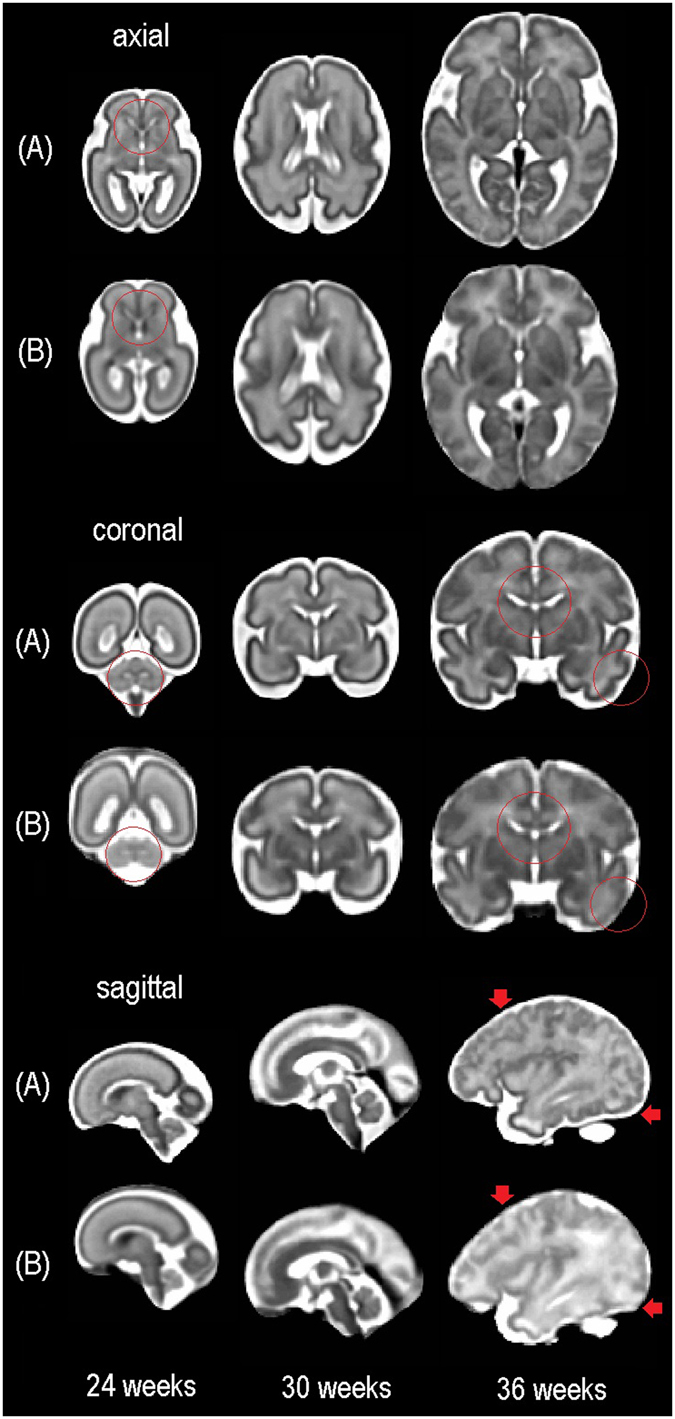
Visual comparison of our spatiotemporal fetal brain MRI atlas (A) and the atlas from brain-development.org (B). We did not register or resample the atlases to avoid artificial blur; we instead tried to compare the closest planes in each view at three ages (24 weeks, 30 weeks, and 36 weeks GA). The images in (B) are generally smoother than those in (A) but lack anatomic details compared to the images in (A). Red circles and markers point at some of the areas with relatively blurred anatomy on the atlas from brain-development.org but with more details on the CRL fetal brain atlas. Both atlases are available online.
